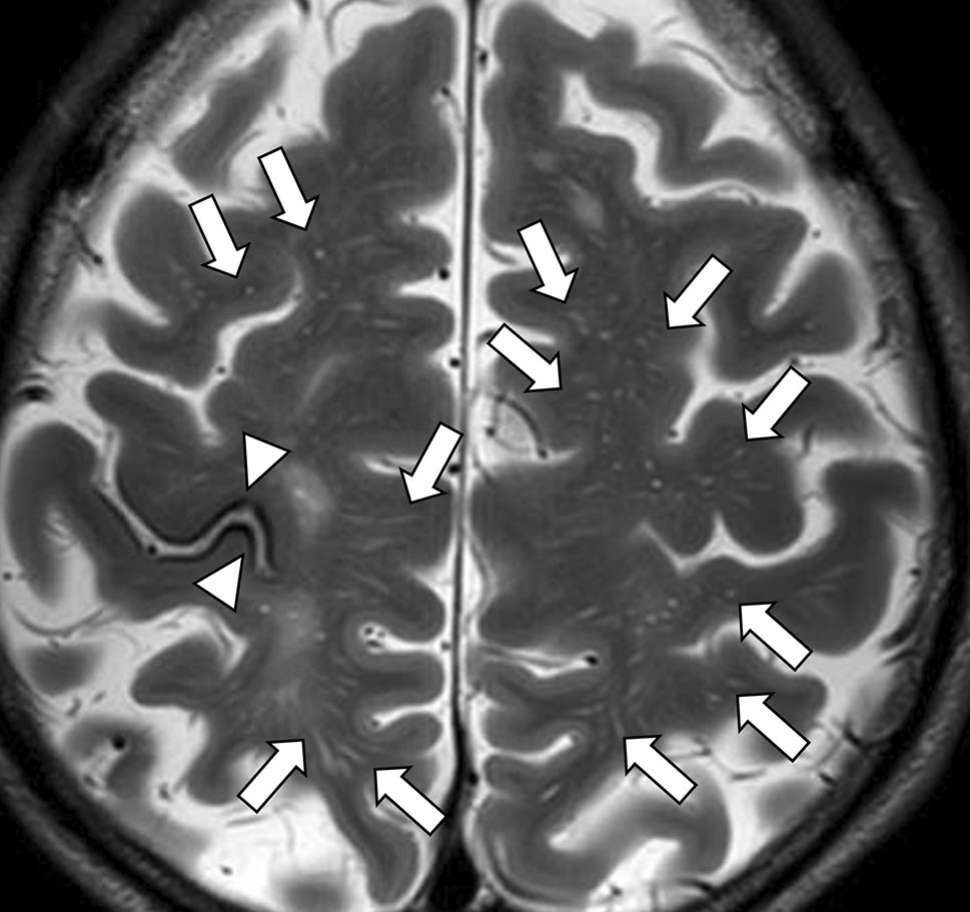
A 71-year-old male with probable CAA. MMSE score is 22. T2-weighted image shows severe PVS-CSO (arrows) in bilateral hemispheres. A hypointensity was observed in the right central sulcus, indicating cSS (arrowheads)
